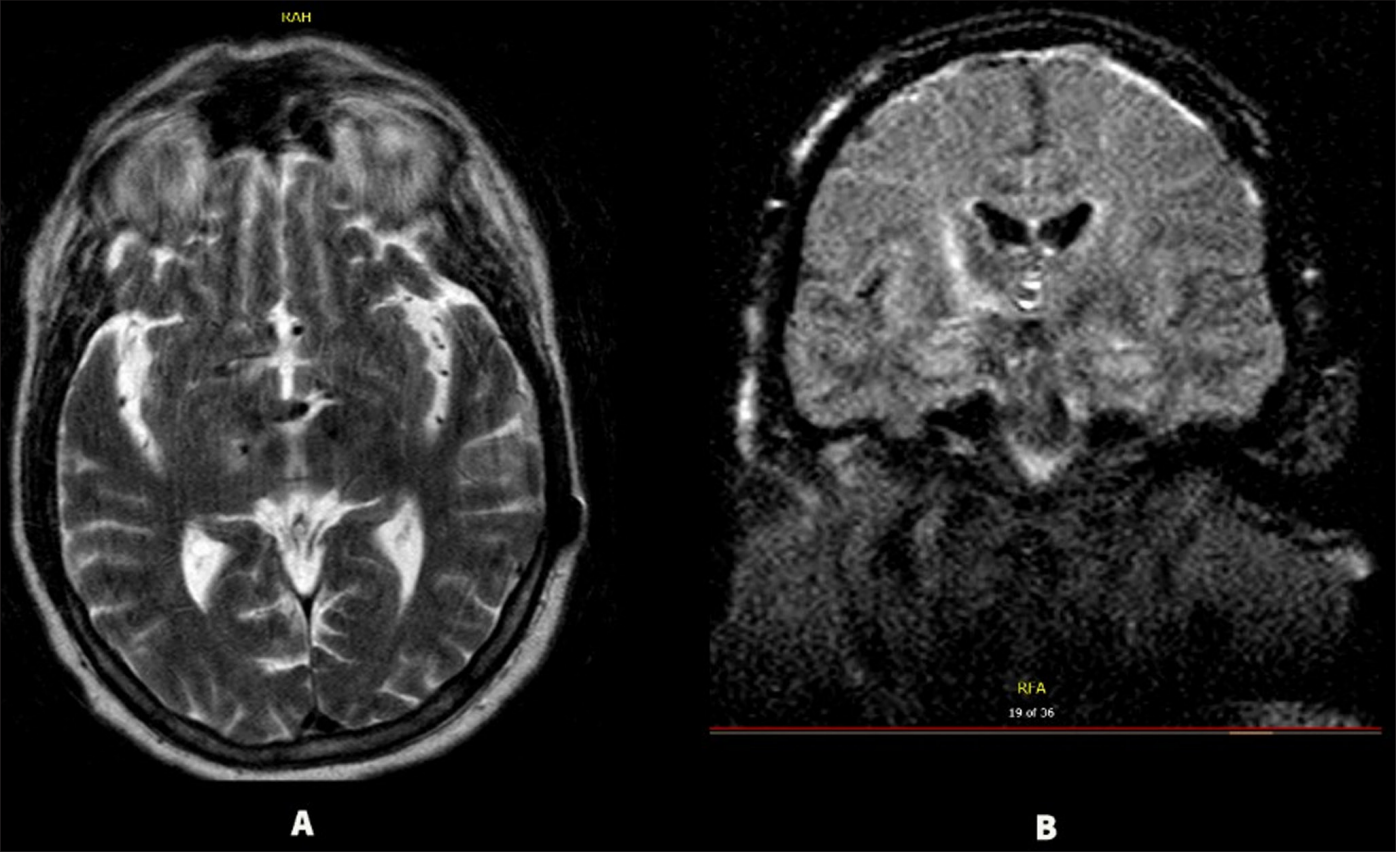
(Case 3) MRI brain showing T2 hyperintensity (A) and FLAIR changes (B) seen around the right electrode compared to his prior imaging with associated restricted diffusion (images not available). The signal abnormalities predominantly involved the right thalamus and basal ganglia.
